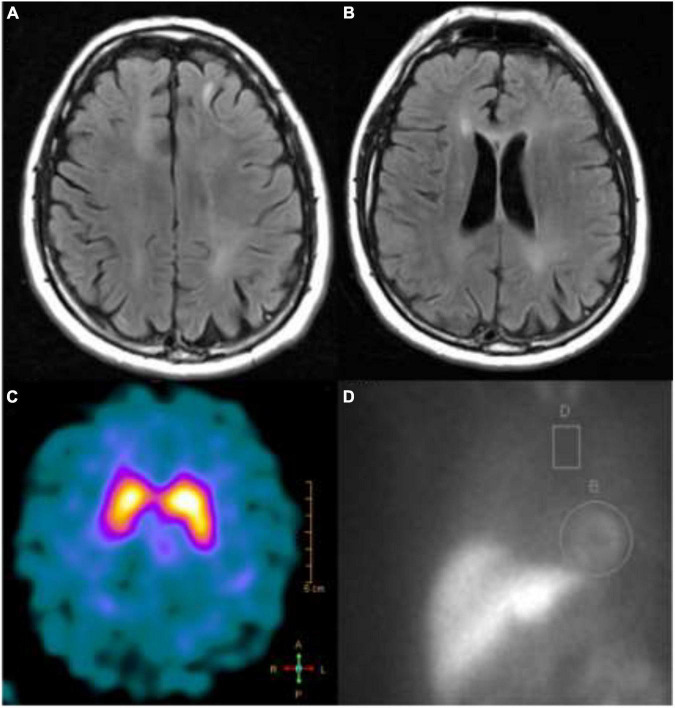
T2-FLAIR MRI showing (A) left frontal juxtacortical hyperintensity and (B) periventricular white matter hyperintensities; (C) a dopamine transporter scan showed reduced tracer uptake in the right putamen; (D) an MIBG scan showing higher than normal (>4:1) heart to mediastinum ratio.
